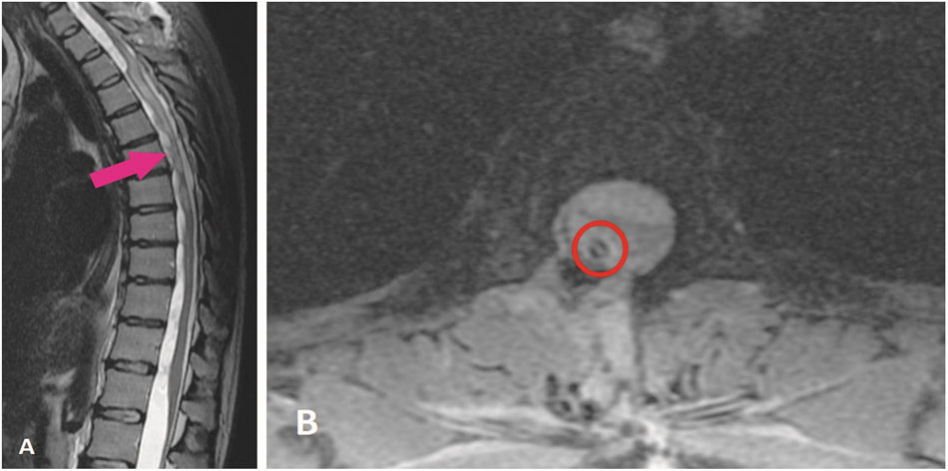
Postoperative T2 sequence MRI images in the (A) sagittal axis and (B) T2 merge axial images after right sided laminotomy, arachnoidolysis, and placement of a cysto-subdural shunt (red circle) at the T2-T3 level posterior to the spinal cord. Improvement of CSF circulation and marked reduction of spinal cord oedema can be visualized (pink arrow).
